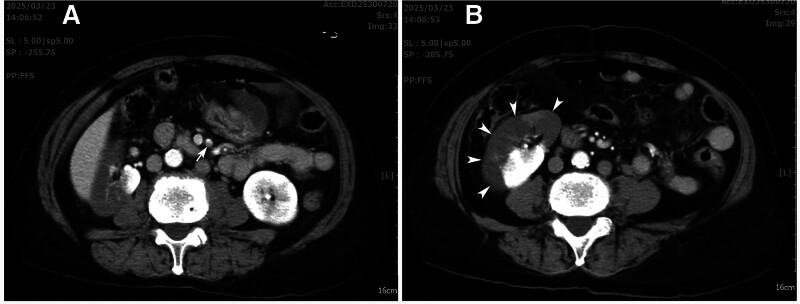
Enhanced abdominal computed tomography showing (A) a filling defect inside the superior mesenteric artery (arrow), and (B) an extensively infarcted right kidney (arrowheads).
